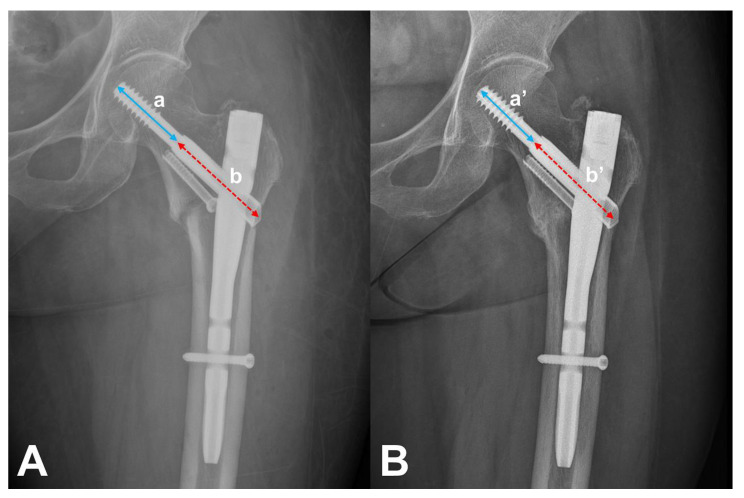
Measurement of sliding distance. The real length of each value (a, a’) was calculated using real length of barrel (b, b’) by proportional expression. Then, sliding distance was measured using immediate postoperative (A) and final radiographs (B) (a^R^ – a’^R^).
